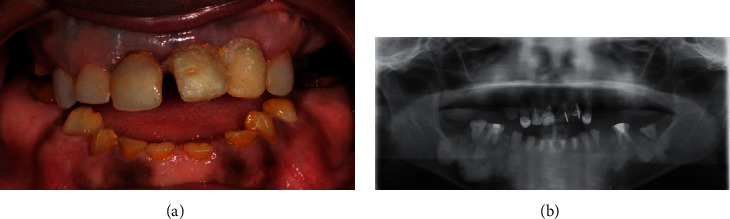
(a) Clinical view of the proband's mother during the first examination. On the upper jaw, all teeth are covered by prosthetic elements. On the lower jaw, the remaining teeth present severe wear with >50% loss of tooth structure. The enamel is orange-brown. (b) Panoramic radiograph of the proband's mother showing mandibular high bone density, missing teeth, absent enamel, and many restored and endodontically treated teeth.
